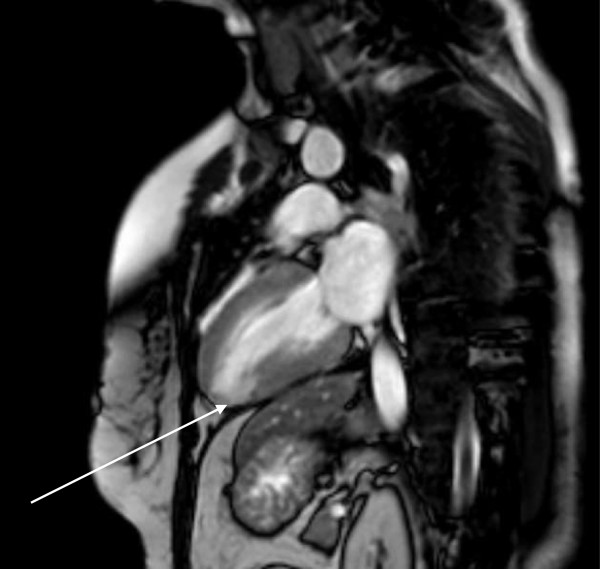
Cardiac magnetic resonance imaging after fourth event of TTC: transmural scar tissue (inferior apical; white arrow).
